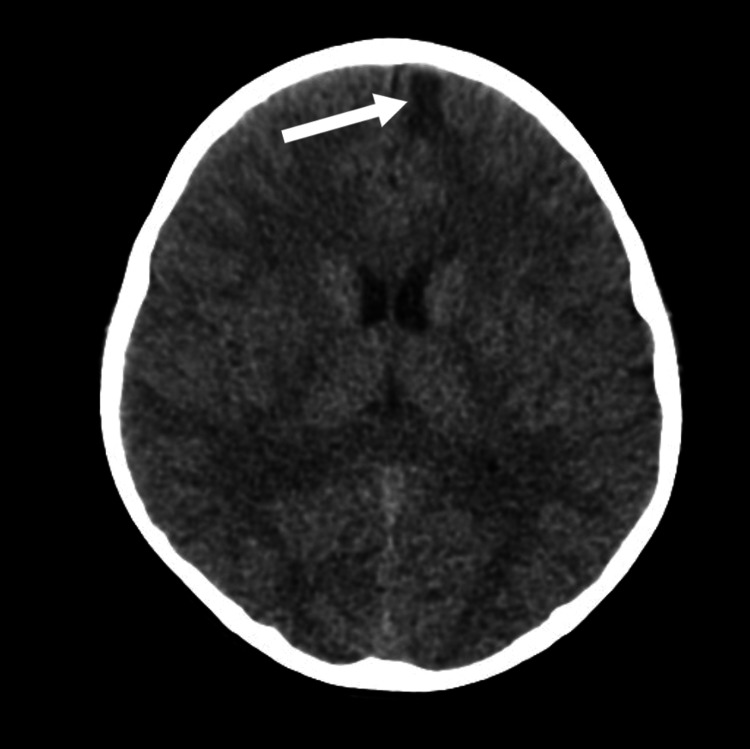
CT brain without contrast CT brain without contrast showing a small (1.8 × 1.3 cm) white matter hypodensity with relative sparing of the cortical margin, noted in the left frontal lobe (arrow). No evidence of volume loss is seen. No obvious mass effect or midline shift. CT: computed tomography
